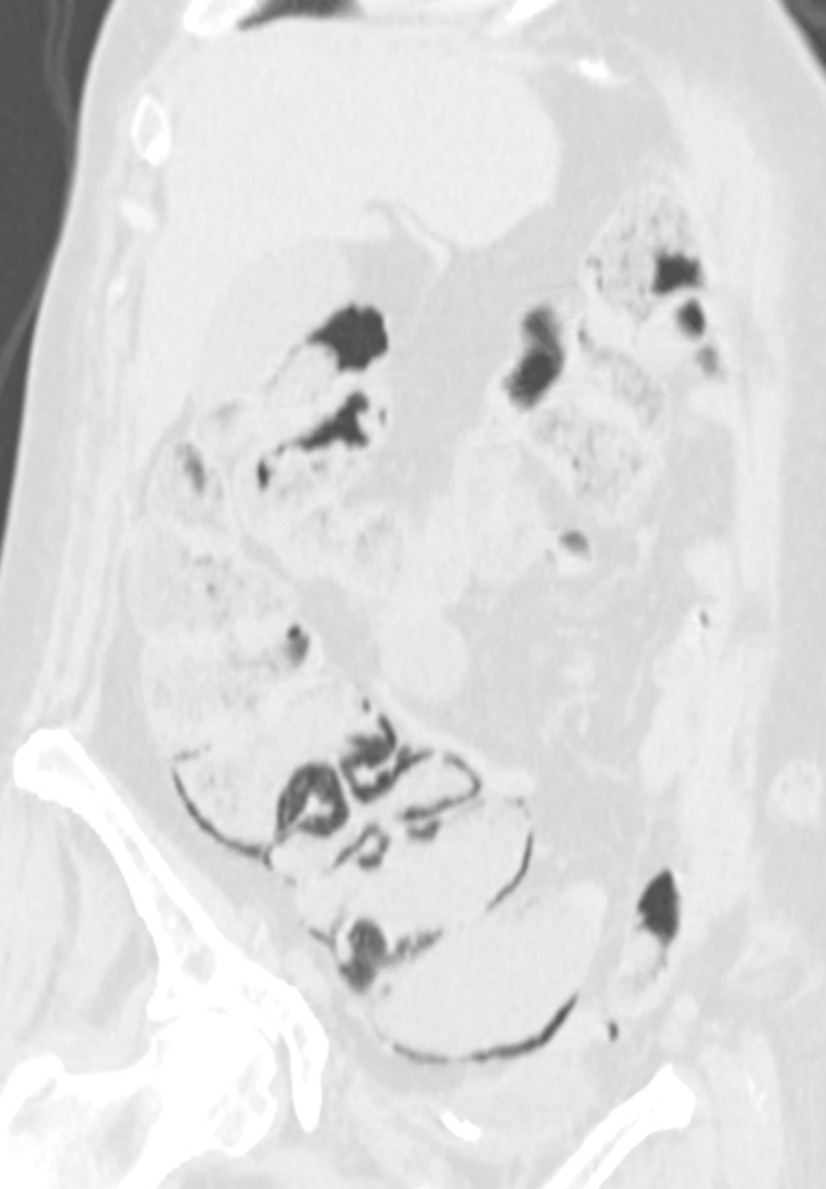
Case 4. A 57-year-old female receiving chemotherapy, including cetuximab after surgery, and radiotherapy for colorectal cancer. Restaging total-body CT scan; coronal oblique reformatted image; lung window. Moderate amount of intramural gas involving the caecum and ascending colon with the absence of pathological wall dilatation or thickening.
